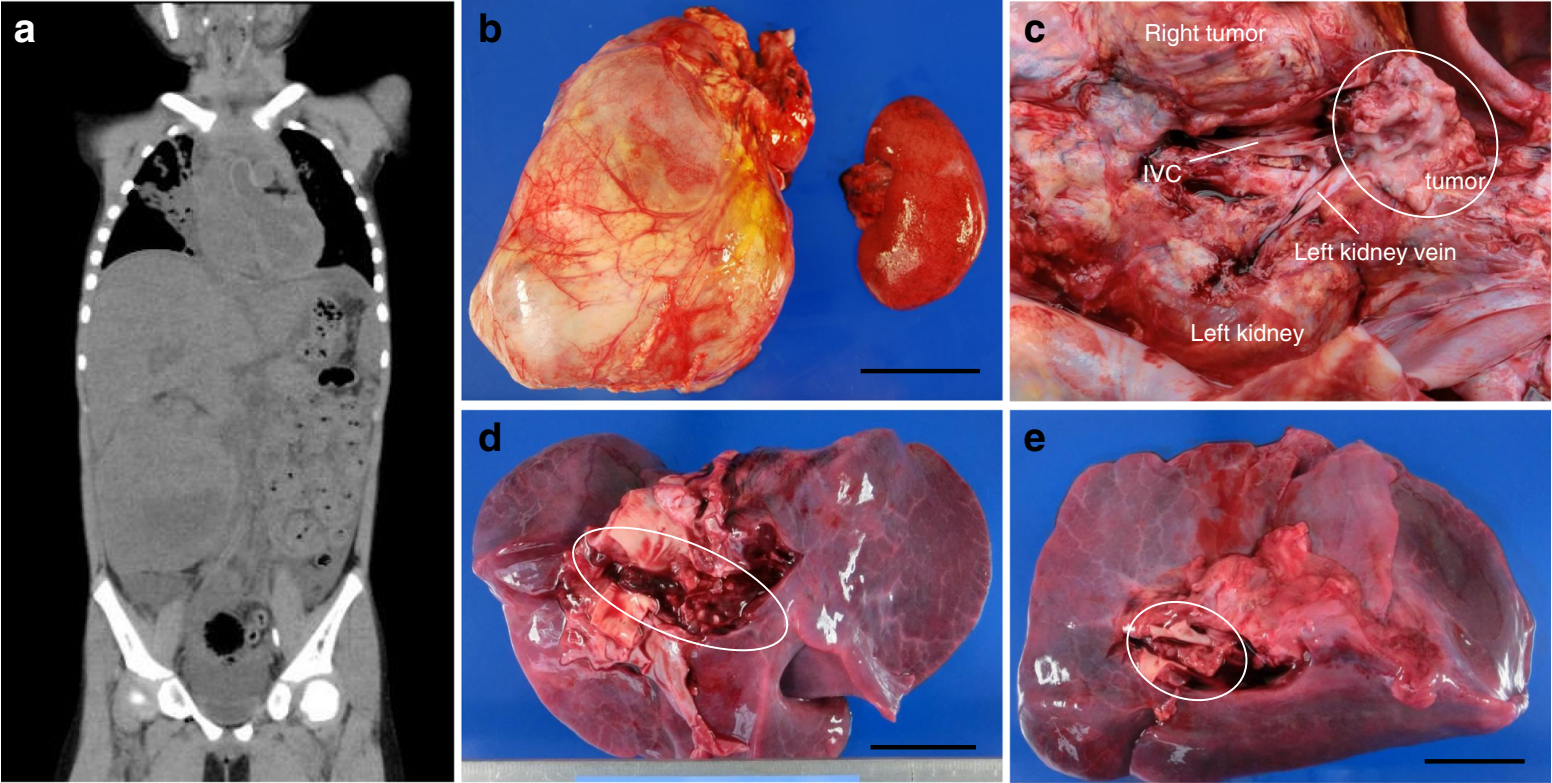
a Plain postmortem CT scan shows a right tumor mass extending into the inferior vena cava (IVC). b Right tumor and normal left kidney are shown. c The tumor extends into the IVC. d Multiple greyish tumors and blood clots are observed in the right pulmonary artery. e Greyish tumor occupies the left pulmonary artery. Black bar represents 5 cm
